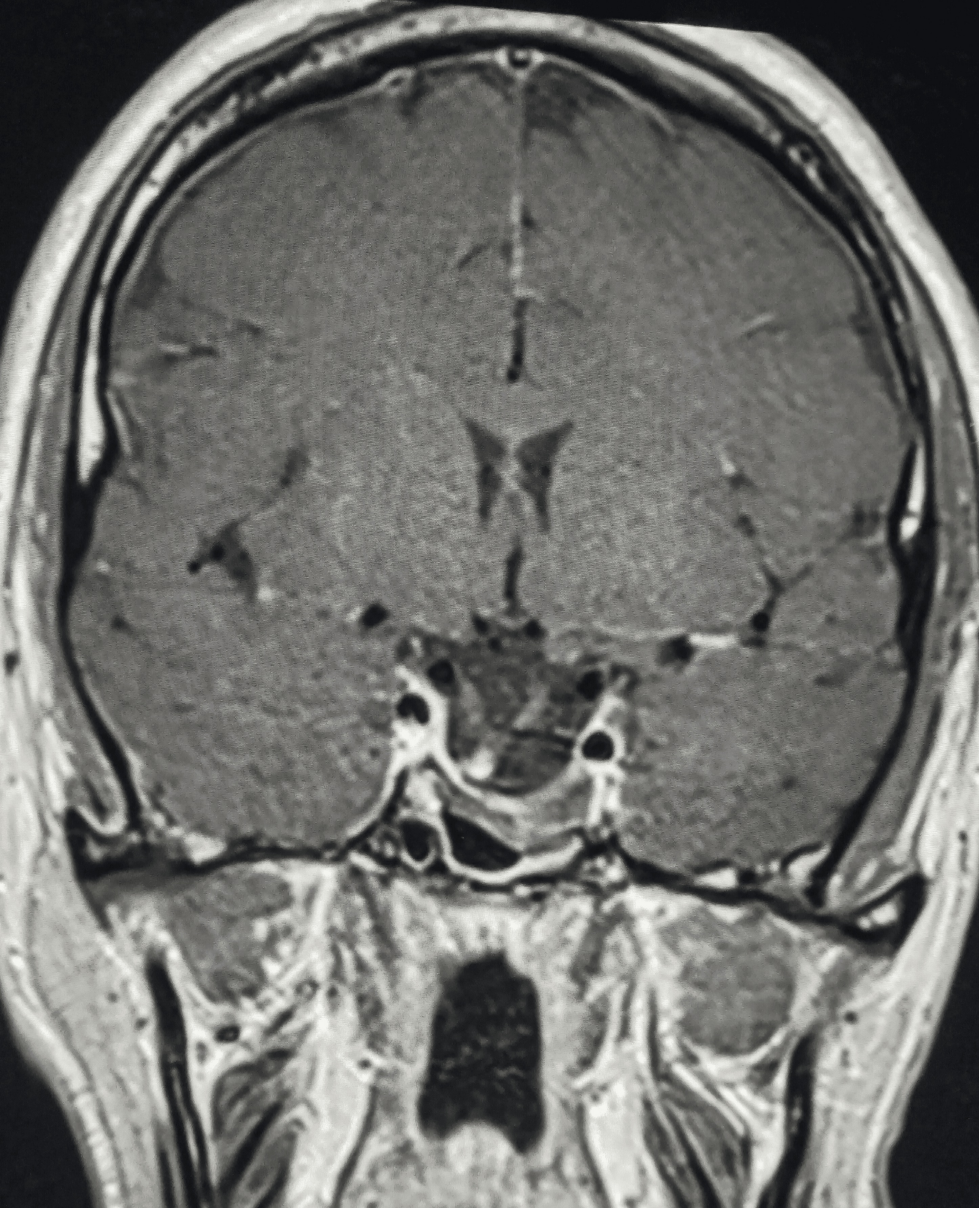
Pituitary MRI - coronal view Twelve months after treatment.
